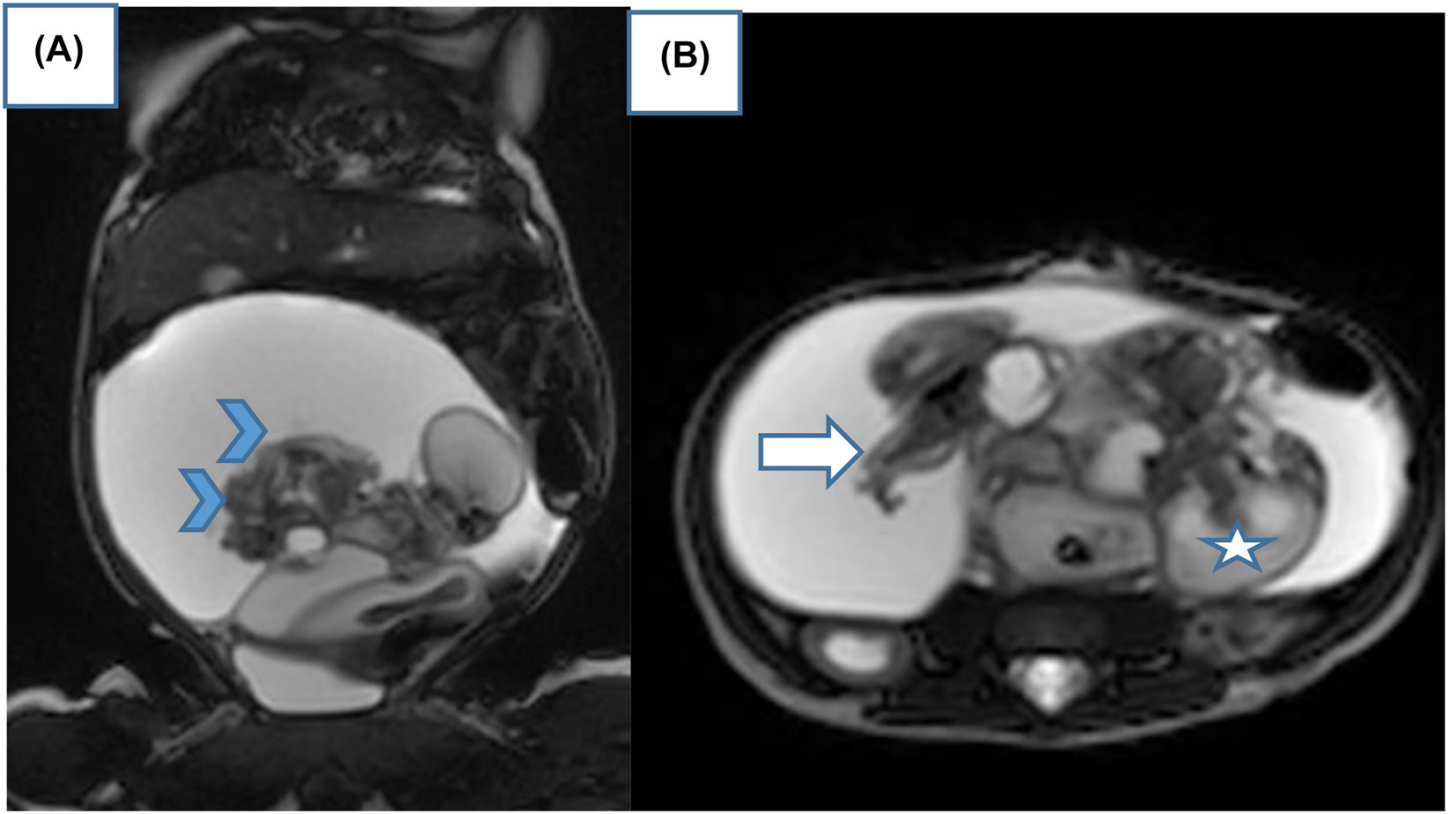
(A, B): Postnatal MRI shows the same feature of US. Heterogeneously cystic mass with calcifications resembling limbs (arrow). It is also contains structures resembling intestines (head arrow) and brain (star).
